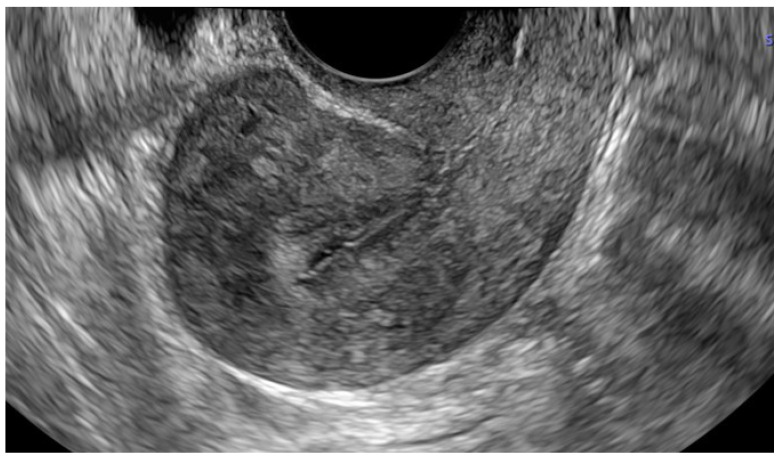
Two-dimensional transvaginal ultrasound image obtained six months after treatment, demonstrating normal cervical and uterine anatomy, with no pathological findings.
